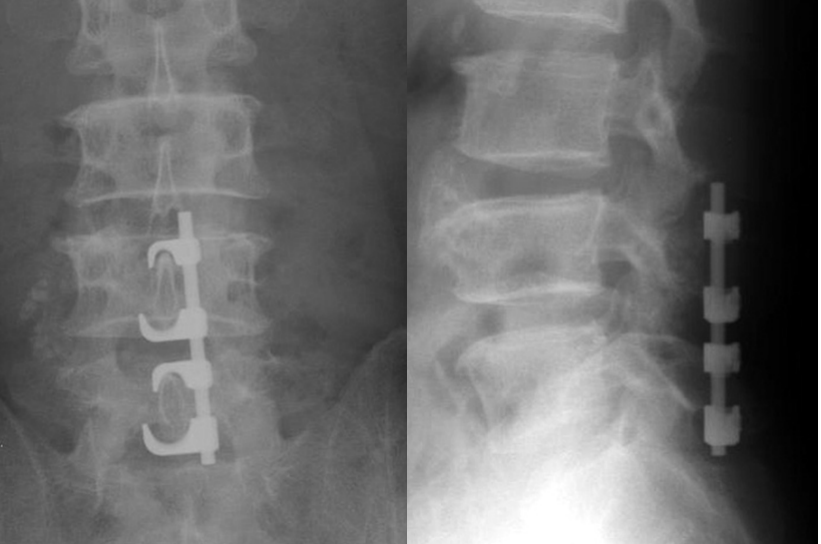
X-rays of a case of single-level fusion
